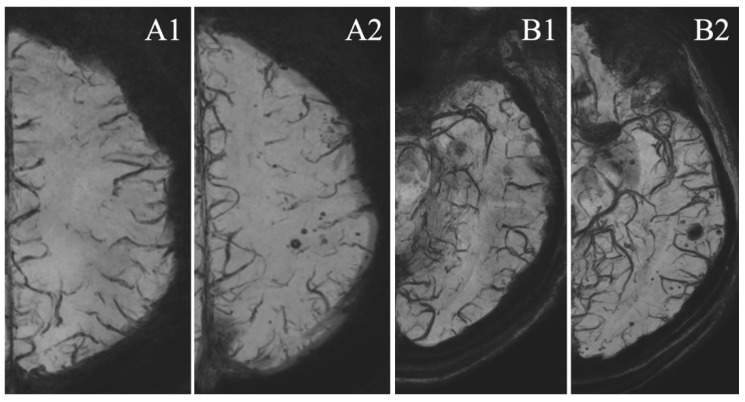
MSA appearance. Axial ten millimeters minimum intensity projection (Mini-IP) reconstructions of magnetic resonance imaging (MRI), susceptibility weighted imaging (SWI) sequence of the same patient. (A1,B1) Pretreatment scans, (A2,B2) follow-up at 36 months after treatment by stent (flow diverter, FD) of a left posterior communicating artery aneurysm. Notice the preferential disposition of MSA in the territory of the stented artery (left internal carotid artery).
